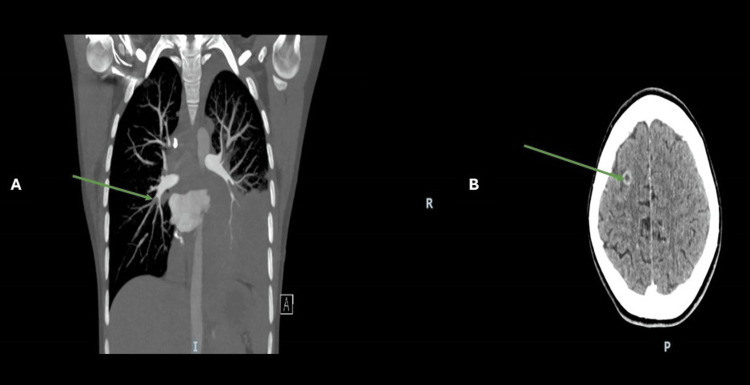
Case 2. Panel A, green arrow: Pulmonary embolism involving the right middle and right lower lobe segmental and subsegmental pulmonary arteries seen in CT pulmonary arteriography (CTPA). Panel B: Brain metastasis seen in head computed tomography. The green arrow demonstrates a 1 cm ring-enhancing intra-axial lesion at the level of the right frontal lobe suggestive of a metastatic lesion. It is surrounded by moderate vasogenic edema.
